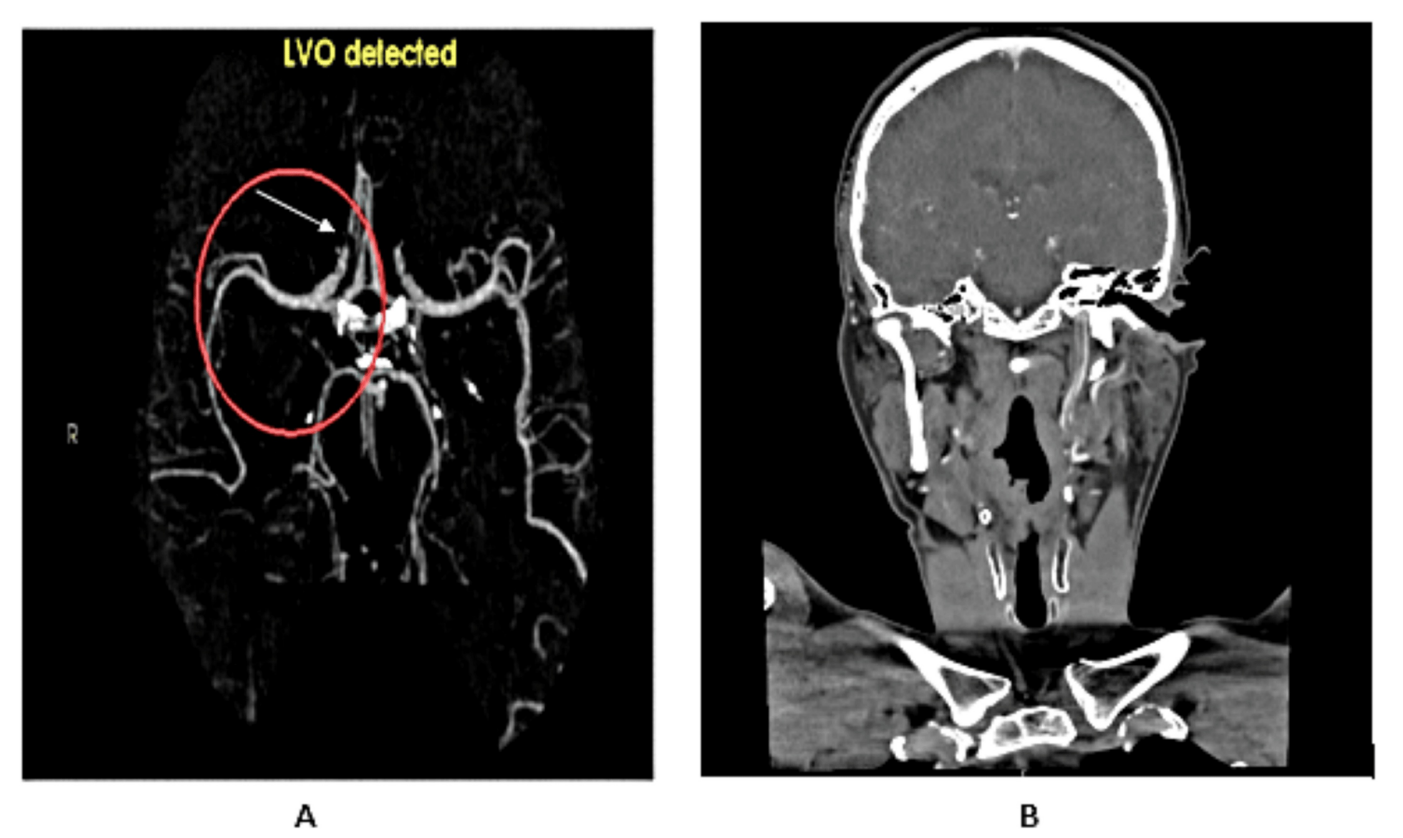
CT angiography of the head and neck showing a large filling defect noted in the left ICA extending 63 mm from its origin to the carotid canal and the base of the skull. These features are highly suggestive of an ICA dissection CT: computerized tomography; ICA: internal carotid artery
